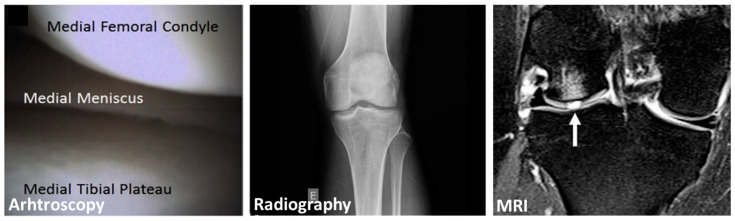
Standard diagnostic methods of KOA. Reproduced with permission from ref. [27] (Arthroscopy), ref. [28] (Radiography), and ref. [29] (MRI). On radiography, “E” is an indicator for “Left”. The arrow points to a subchondral bone marrow oedema-like signal associated with a (grade IV) lateral femoral condyle cartilaginous defect. Reproduced under the Creative Commons License (https://creativecommons.org/licenses/by/4.0/, accessed on 1 March 2025).
